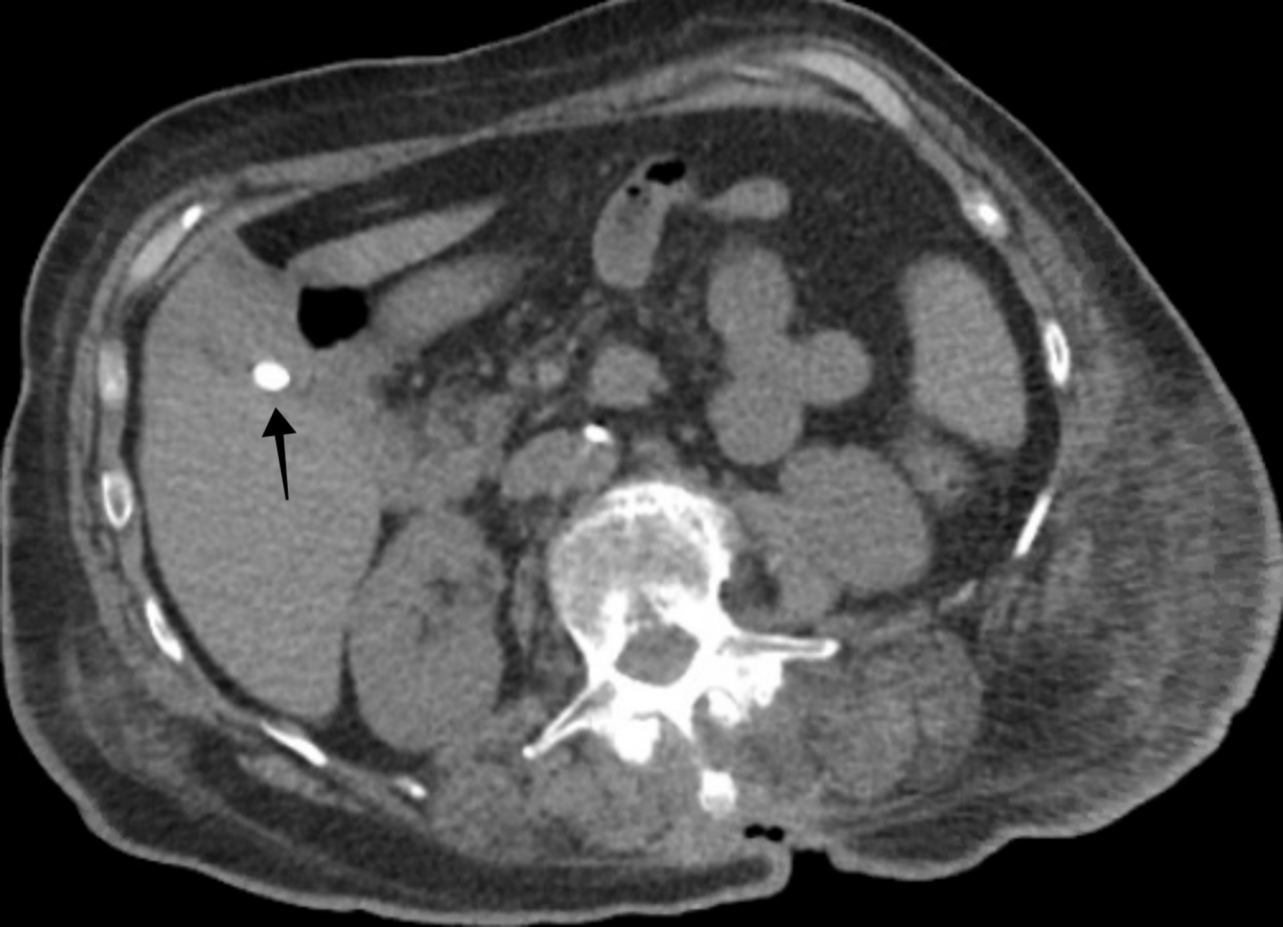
Computed tomography of the abdomen revealing cholelithiasis
